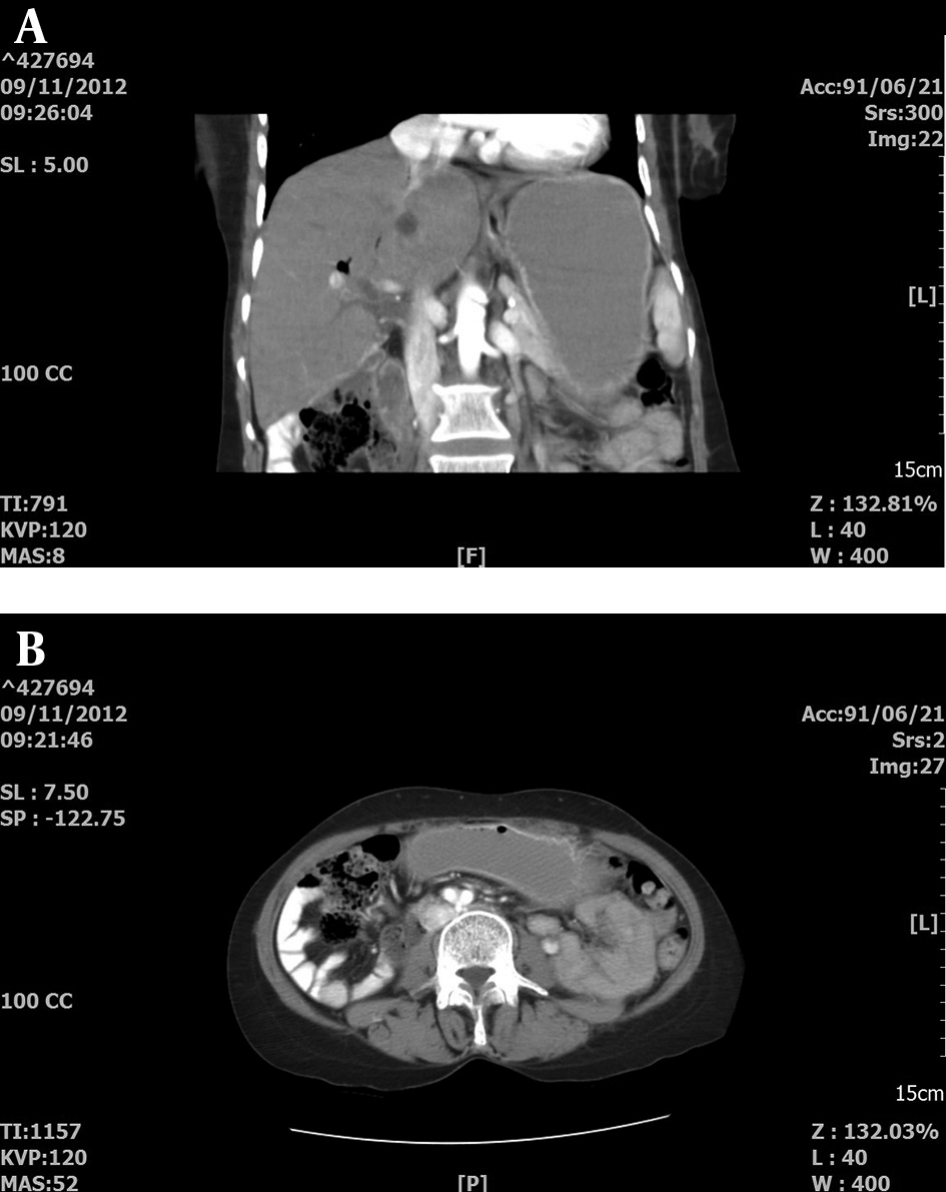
Post Treatment Ct Scan of the Abdomen CT Scan of the Abdomen of the Patient Taken 2 Months After End of Treatment With no Evidence of Active Disease in the Liver (A) and in the Anastomosis Area (B)
